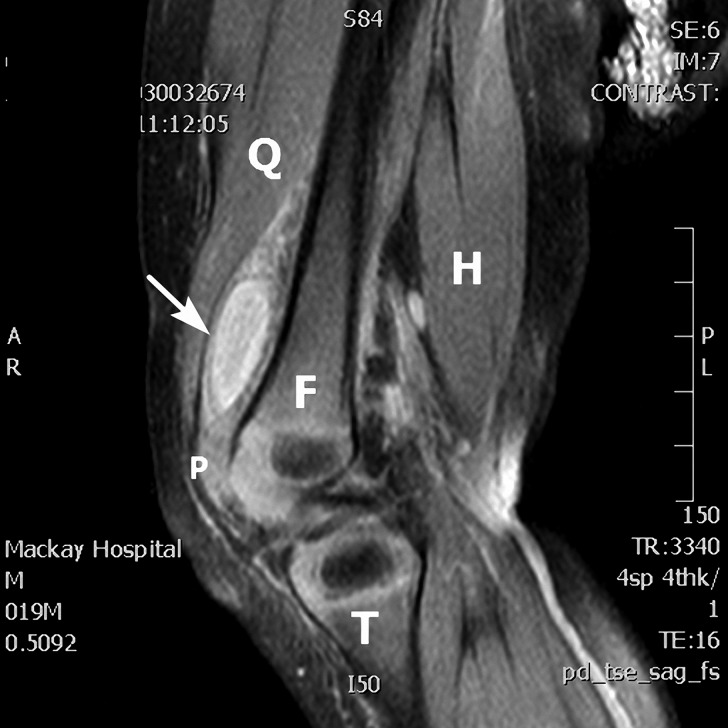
The sagittal view of T2-weighted knee magnetic resonance imaging shows abscess formation in the right suprapatellar bursa (arrow). The anatomic structures are labeled—F = femur, H = hamstring muscles, P = patella, Q = quadriceps muscles, T = tibia.
